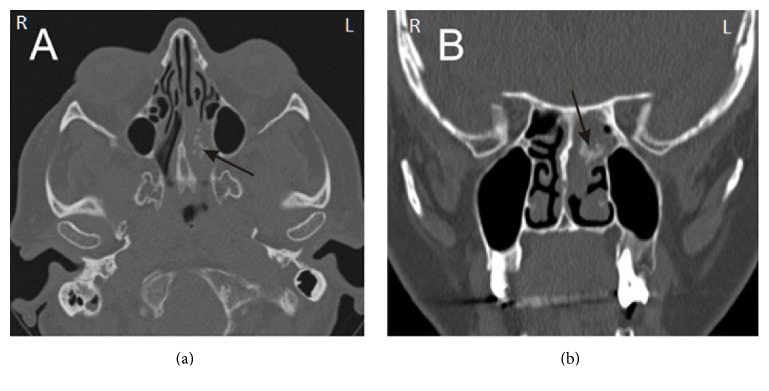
CT scan along the transverse (a) and frontal (b) plane in a patient with inverted papilloma of the nasal cavity and ethmoid cells at the left side; the arrows indicate focal hyperostosis.
